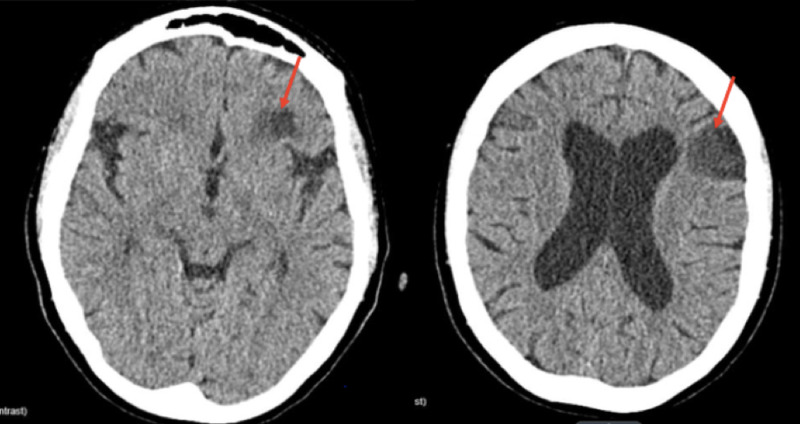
Noncontrast CT of the patient’s head showing hypo-density in the left frontal lobe (red arrows). Abbreviation: CT, computed tomography
